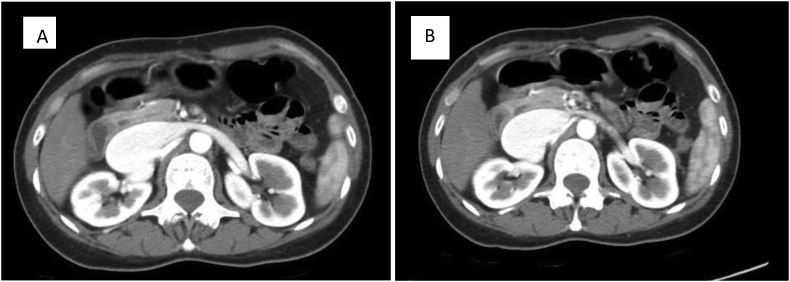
A and B: demonestrate CT angiography images in axial cuts, showing renovascular fistula with dilated contrast filled inferior vena cava.
